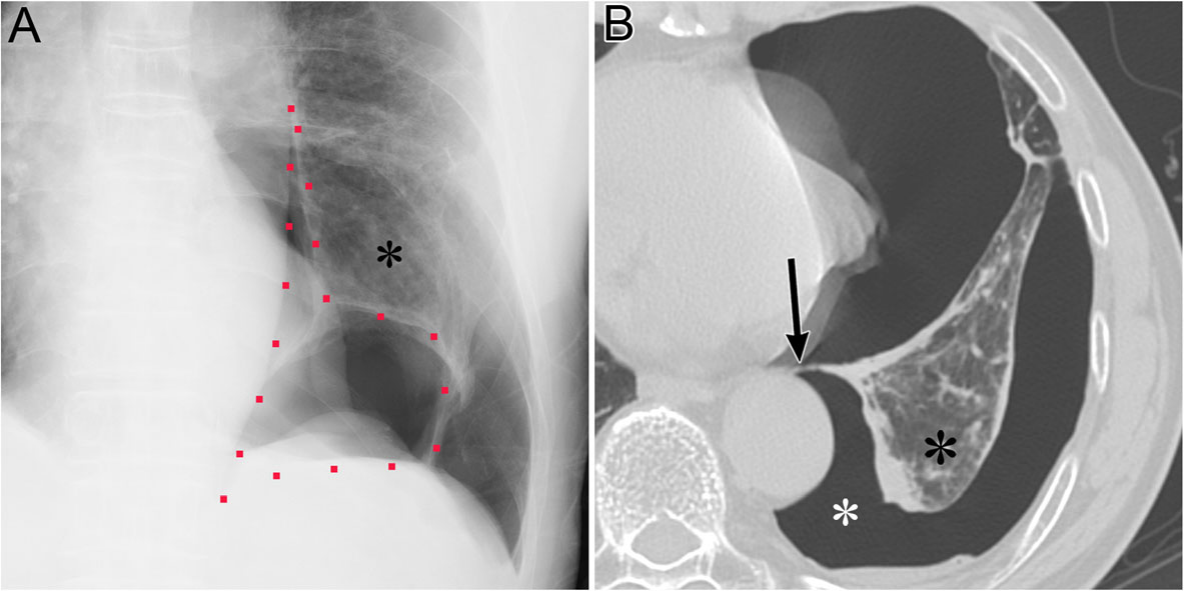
Pneumothorax in the posteromedial space of an 82-year-old man with chronic obstructive pulmonary disease. a A supine radiograph shows a lucent triangle (red dotted line) with a partially collapsed left lower lobe (black asterisk). The lucent triangle corresponds to the air collection space with its vertex in the hilum and a V-shaped base that delineates the costovertebral sulcus between the paraspinal line/descending aorta and the medial surface of the partially collapsed lower lobe. b An axial CT image of the lung bases shows pneumothorax in the posteromedial space (white asterisk). The left inferior pulmonary ligament (arrow), which is the linear structure between the mediastinum and the left lower lobe (black asterisk), are clearly visible
